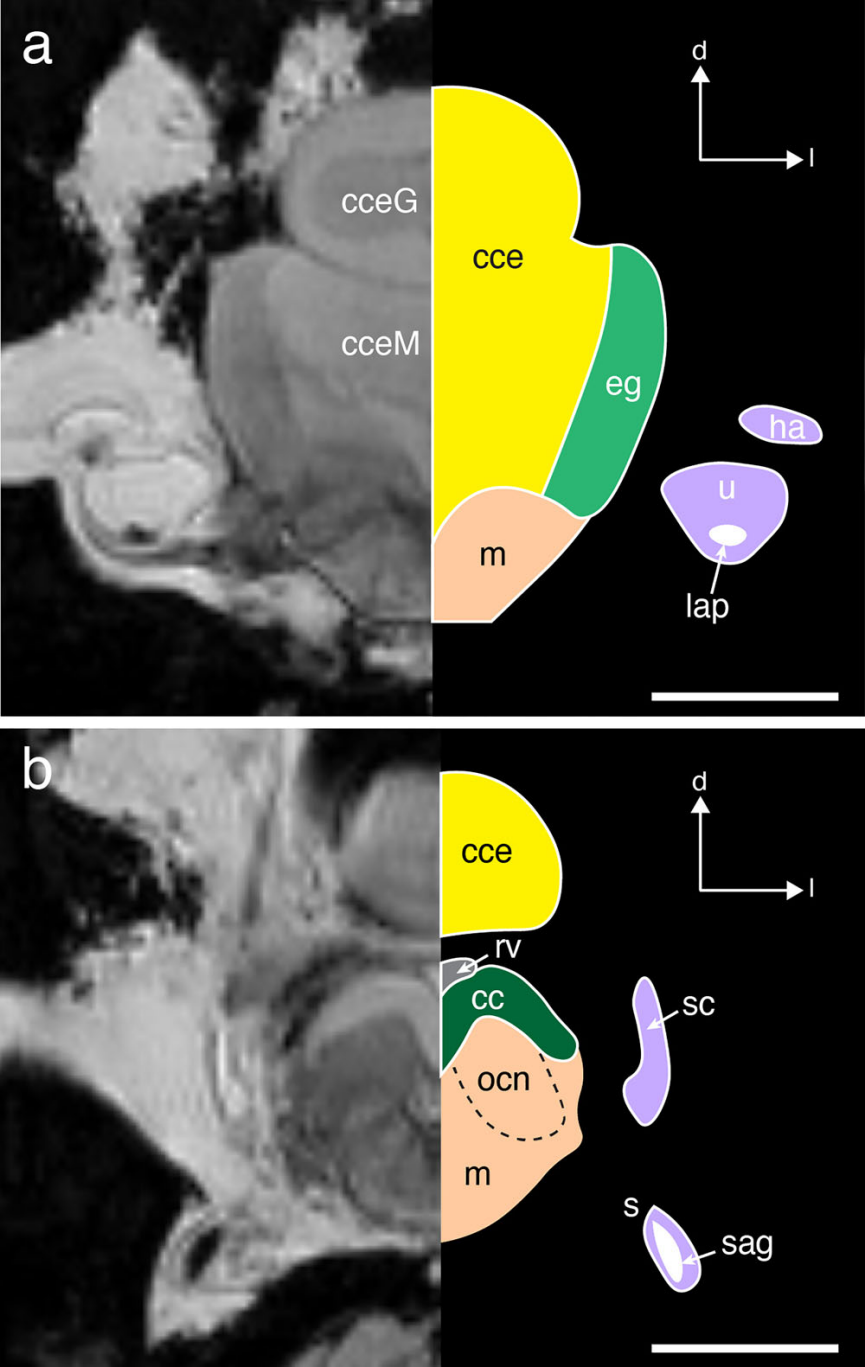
A pair of axial sections through the anterior part (a) and the posterior part (b) of hindbrain of *T. maccoyii*. The MRI image is on the left, and line tracings on the right indicate different regions. Each region is colored with colors corresponding to the 3D segmentations, while the ventricle is rendered in gray. Inner ear structures are colored with light purple. Dashed line indicates the likely boundary. The membranous structures of the inner ear: horizontal ampulla (ha), semicircular canal (sc), saccule (s), and utricle (u), and the calcified otoliths: sagitta (sag) and lapillus (lap) are also visible laterally adjacent to the brain. Orientation: d, dorsal; l, lateral. cc, cristae cerebelli; cce, corpus cerebelli; cceG, granular layer of the cce; cceM, molecular layer of the cce; eg, eminentia granularis; m, medulla; ocn, octavolateral nucleus; rv, rhombencephalic ventricle. Scale bars, 5 mm (a, b).
